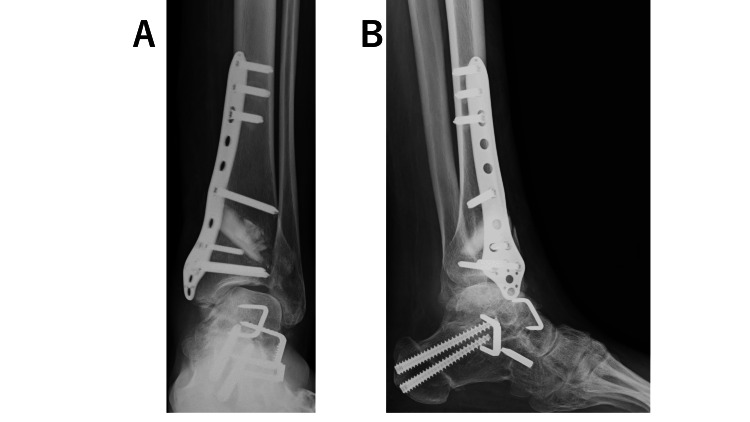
Plain radiographs of the left foot after the revision surgery. (A, B) Range of motion at the ankle joint was maintained and the stability was good.
